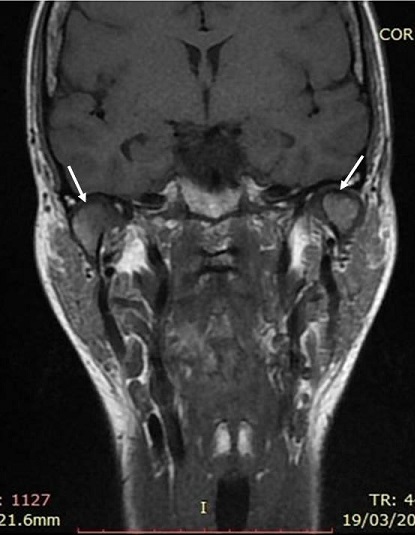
Magnetic resonance imaging (MRI) coronal slices showing bilateral subchondral geodes and thinned cortical of mandibular condylar process
